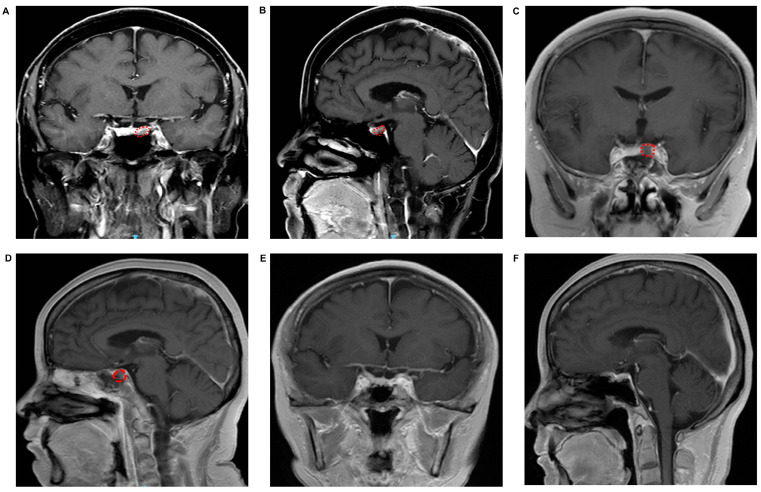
Preoperative and postoperative MRI. (A,B) Coronal and Sagittal MRI scans show a microadenoma adjacent to the left cavernous sinus. (C,D) Coronal and Sagittal MRI scans taken 2 days after surgery, indicating that the tumor was removed completely. (E,F) Coronal and Sagittal MRI scans taken 3 years after surgery, indicating no recurrence.
